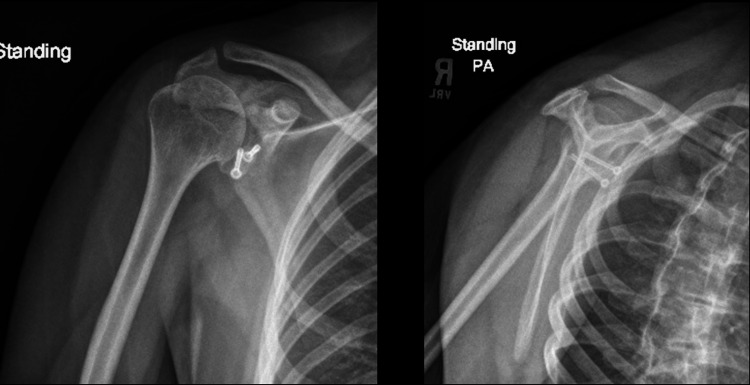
Radiographs of the right shoulder at five-week follow-up from Case 2
